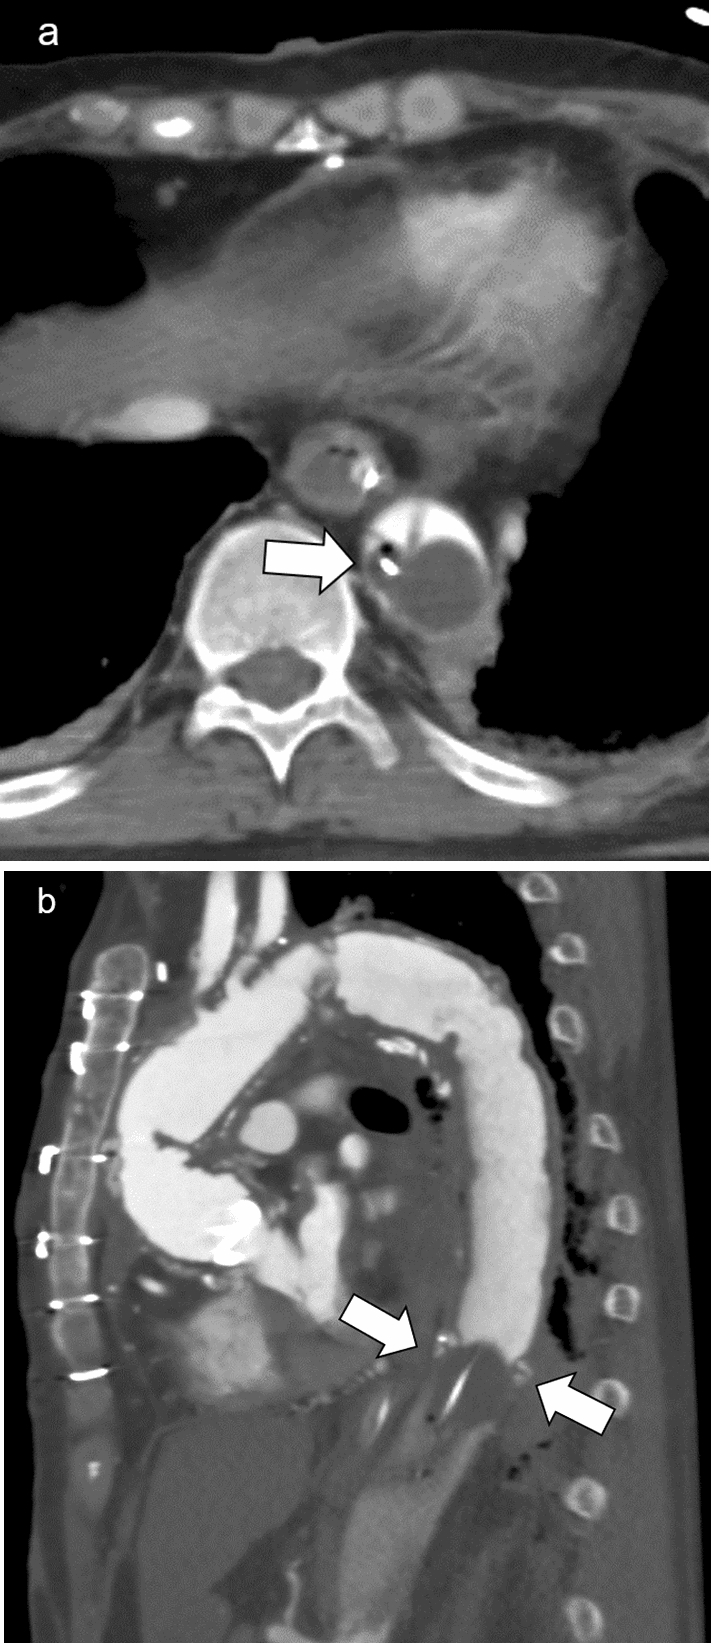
Thoracic contrast-enhancement computed tomography (a: axial image, b: sagittal view) in a patient with prior aortic replacement for aortic dissection (Stanford type A) showing that the catheter shaft was positioned in the smaller lumen (true lumen) (a, arrow) and the balloon was inflated in the false lumen through the double-barreled anastomosis (b, arrows)
